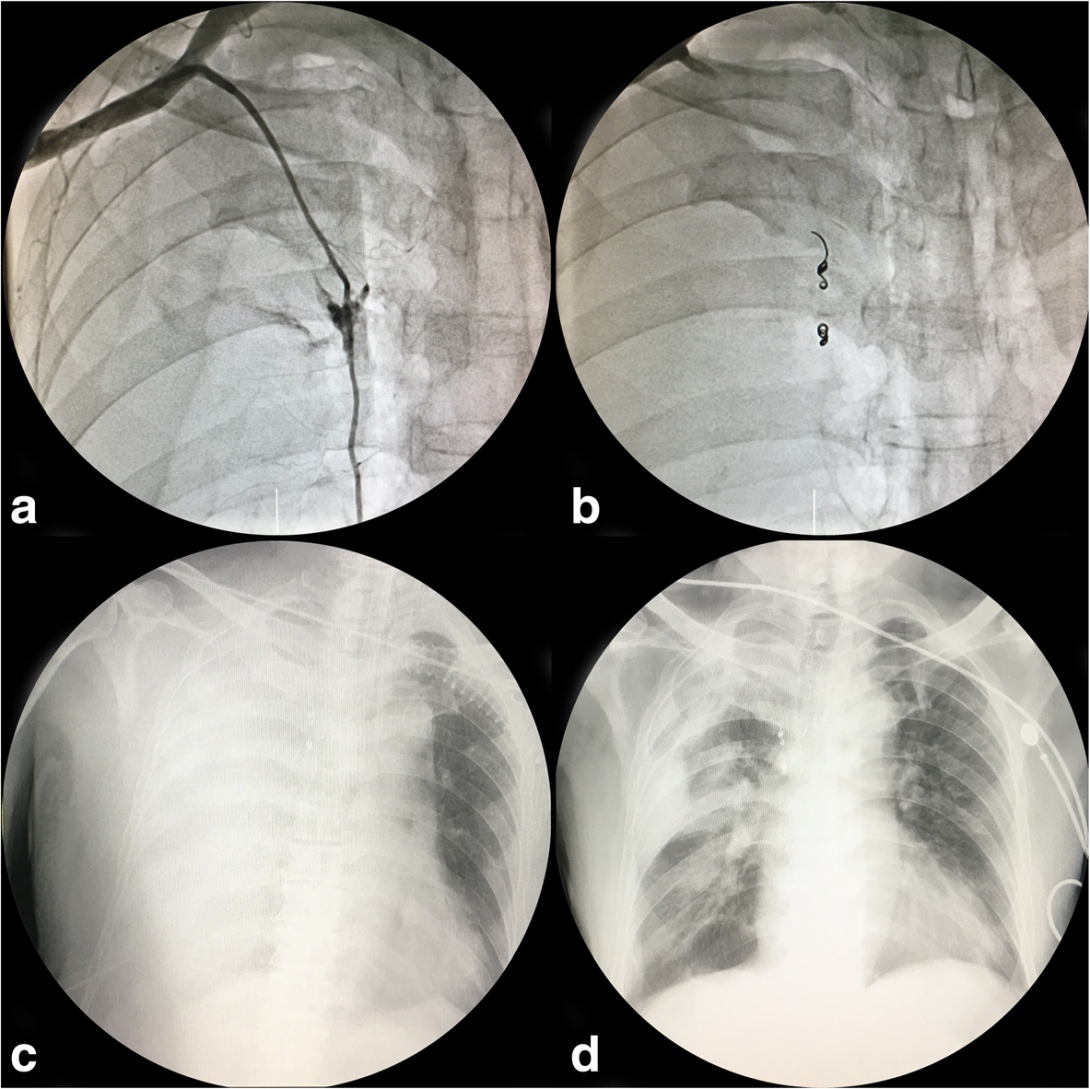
IMA angiography shows exudation in the third rib segment of the right IMA (a) The hemorrhage was staunched by embolization with coils (b). Chest radiograph shows a large shadow in the right chest suggestive of a pleural haemothorax (c). The pleural haemothorax is significantly reduced after treatment (d)
